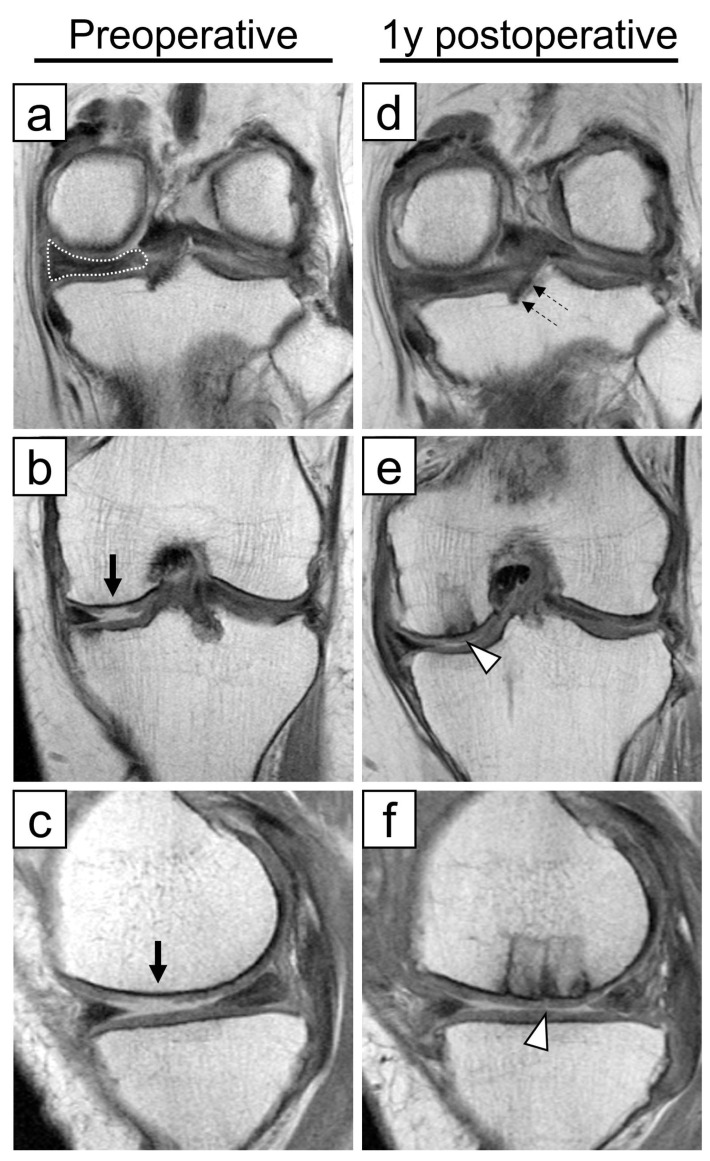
Preoperative and postoperative magnetic resonance imagings of a representative case: (a,b,d,e) coronal views; (c,f) sagittal views. A 55-year-old woman injured her left knee by twisting it on stairs. Preoperative MRI revealed a medial meniscus posterior root tear (a) (“giraffe neck” sign; white dotted area) and focal cartilage damage on the medial femoral condyle, approximately 2.2 cm^2^ (b,c) (black arrows). Postoperative MRI demonstrated successful repair of the posterior root, with continuity confirmed (d) (dashed arrow) and good incorporation of the osteochondral autograft transplantation (e,f) (white arrowhead).
